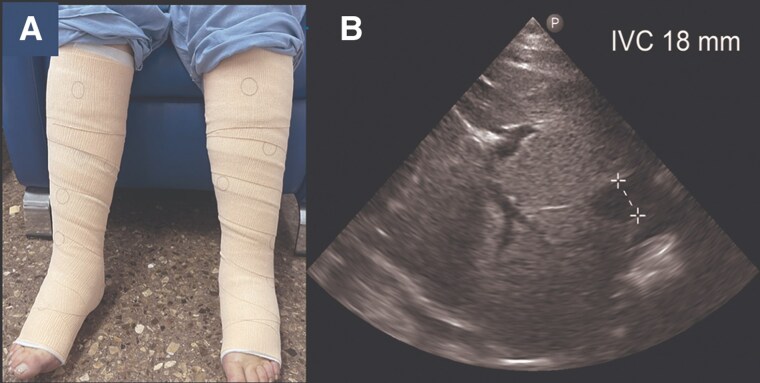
Application of venous leg compression. (*A*) Compression bandage applied to lower limbs. (*B*) Inferior vena cava echocardiography after 2 h of venous leg compression: increase to 18 mm indicating improved intravascular filling.
